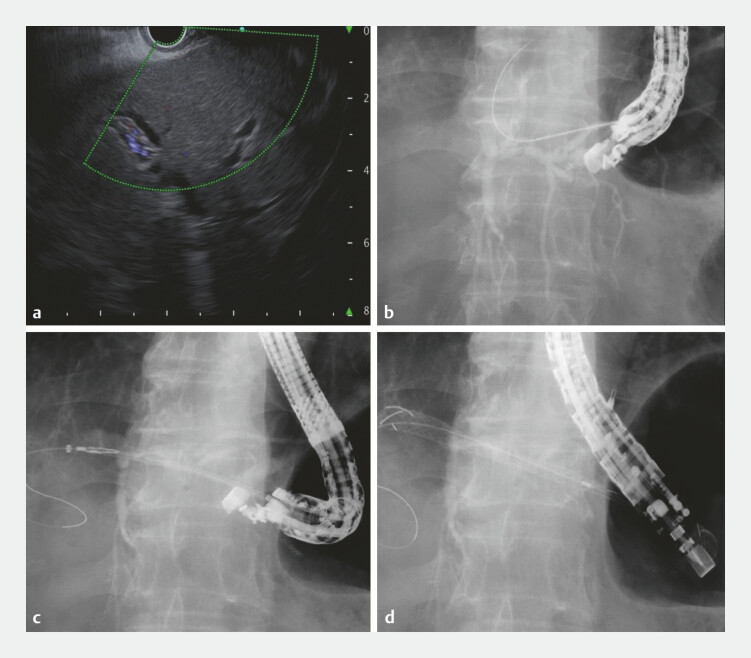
Endoscopic ultrasonography-guided hepatogastrostomy.
